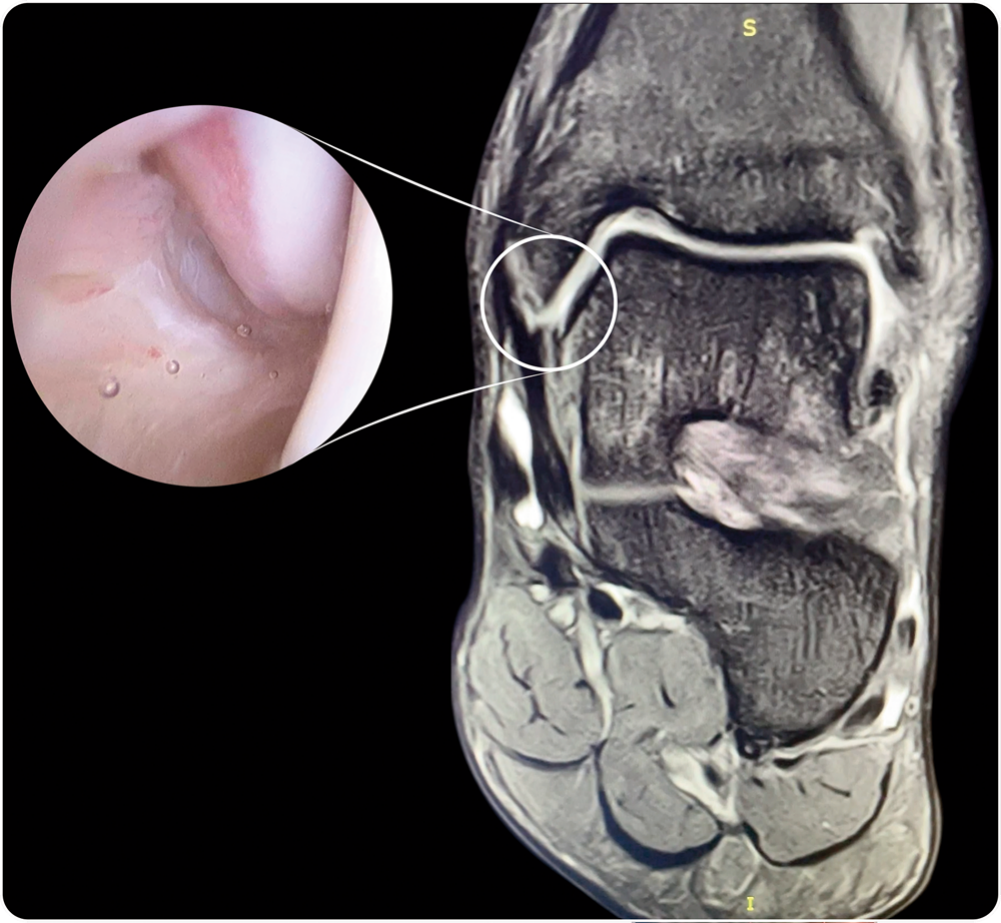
Magnetic resonance imaging showing proximal detachment of the deep fascicle at the level of the anterior colliculus. Arthroscopic view of the deltoid injury.
